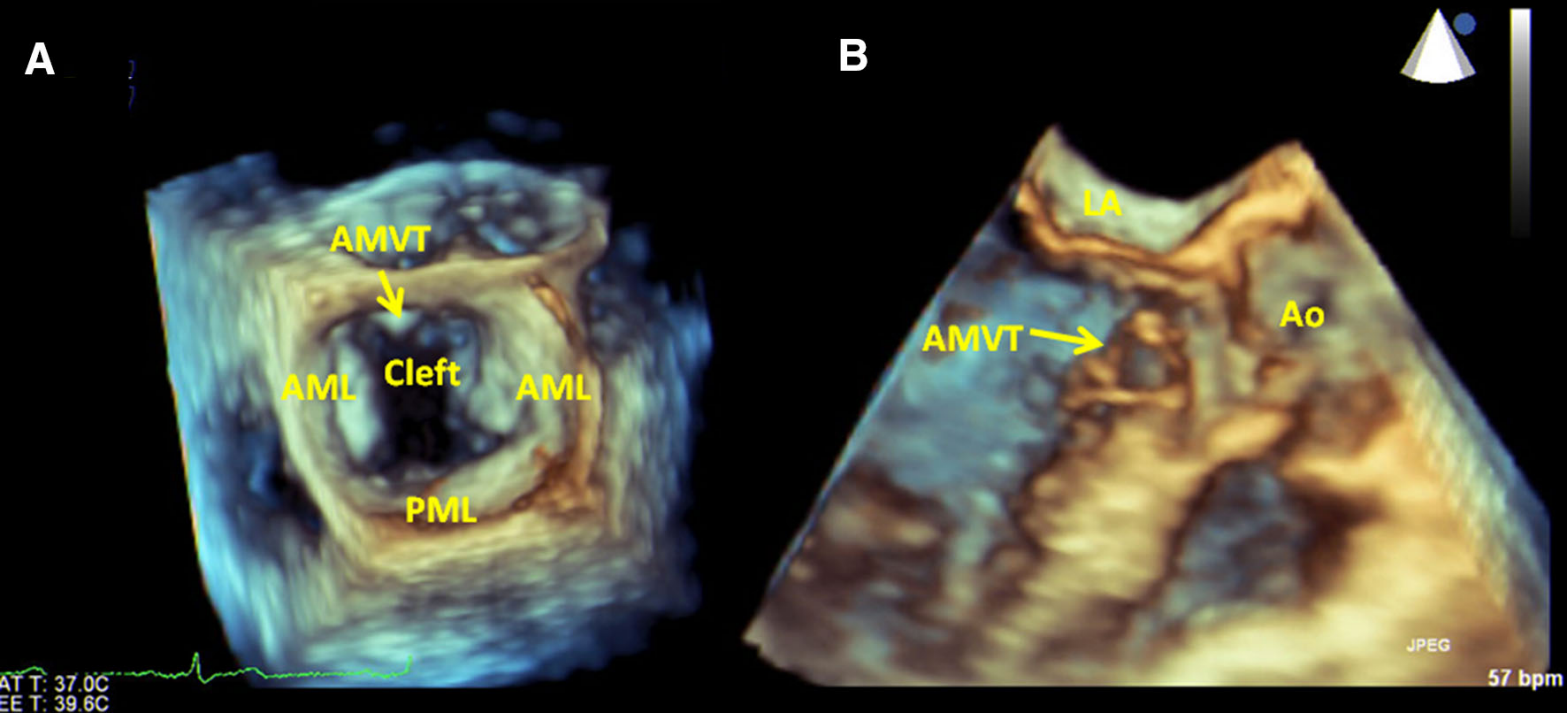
a Three-dimensional transesophageal echocardiography shows in diastole show mitral valve leaflet cleft and AMVT (*arrow*) attached from base of intraventricular septum to anterior mitral leaflet. b Three-dimensional transesophageal echocardiography in the long-axis view shows accessory mitral valve tissue (AMVT) (*arrow*) into the LVOT
